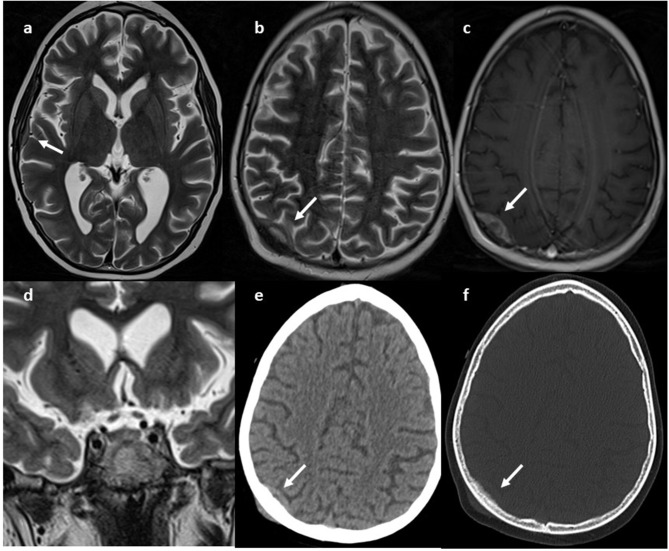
MRI. Axial (a,b) and coronal (d) T2w and axial GdT1 (c) images show two skull lesions located in the right parietal and temporal region, with intense contrast-enhancement. Pituitary gland was normal (e, white arrow) CT axial images (e,f) show infiltrative aspect of the lesion.
